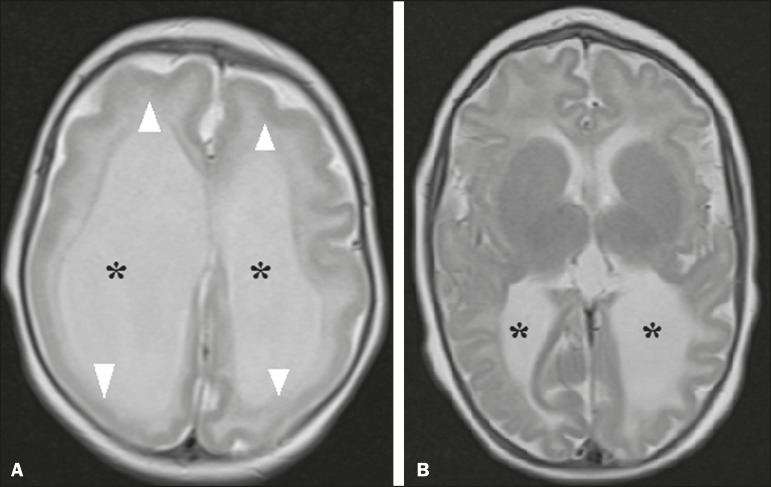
A: A 7-month-old patient. Magnetic resonance imaging, T2, axial section
